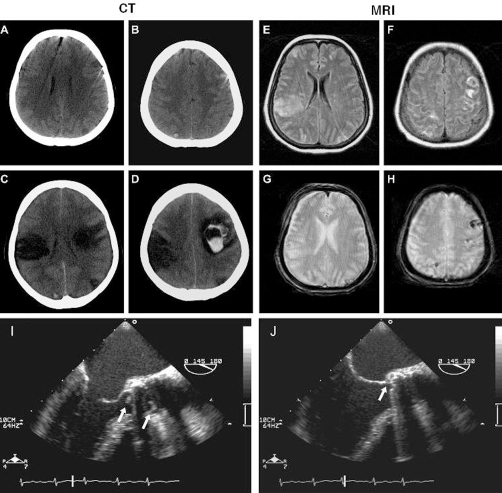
Axial cNECT and cMR images on admission (A+B, E-H) and 4 days after onset of neurological symptoms (C+D). (A+B) NECT images show initially small cortical bleedings left frontal and right occipital and swollen cortex sections right occipital. (C+D) 4 days later the haemorrhage is enlarged, and multiple zones of infarction are visible. (E+F) Axial FLAIR weighted images demonstrate bilaterally multiple cortical and subcortical signal hyperintensities representing multiple ischemic lesions. (G+H) Axial T2*GRE MR images show microbleedings and haemorrhages within infarcted lesions. Transoesophageal echocardiogram examination shortly after admission (I+J). (I) Demonstration of two large oscillating vegetations (arrows), one of 4.5 × 2 mm on the upstream side and one of 4.3 × 7.4 mm on the downstream side of the bileaflet tilting disk valve. (J) Closer examination of the mechanical aortic valve shows relevant thickening of the aortic root indicating an evolving ring abscess (arrow).
